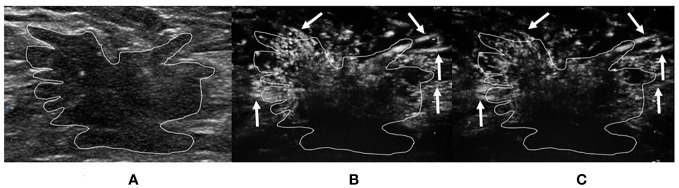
A 40-year-old woman with invasive breast carcinoma proven by pathology. The tumor region is marked in white. (A) Grayscale ultrasound image of breast tumor. (B) The ≤30-s phase: microvasculature morphology shows a crab claw-like pattern (arrows) in the peripheral region of the tumor. (C) The >30-s phase: microvasculature morphology shows a crab claw-like pattern (arrows) in the peripheral region of the tumor.
